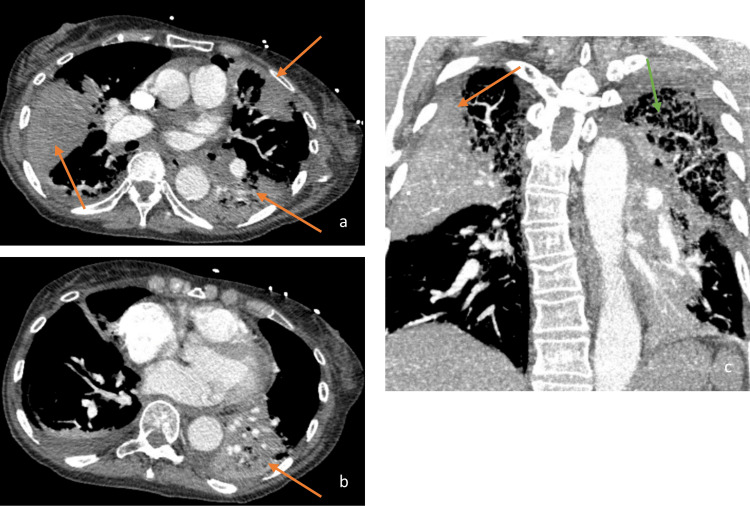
Transverse (a, b) and Coronal (c) Views of a Computed Tomography Angiography Scan of the Chest. The Scan Demonstrating Multifocal Opacities Within the Right Middle & Superior Left Lower Lobes of the Lungs (orange arrows) and Emphysematous Changes (green arrow).
